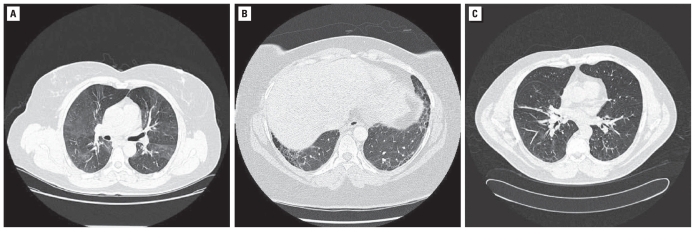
Representative HRCT images. (*A*) Air trapping (mosaic pattern) in Patient D (end expiratory). (*B*) UIP-like pattern in Patient E. (*C*) Sarcoid-like pattern in Patient G.
